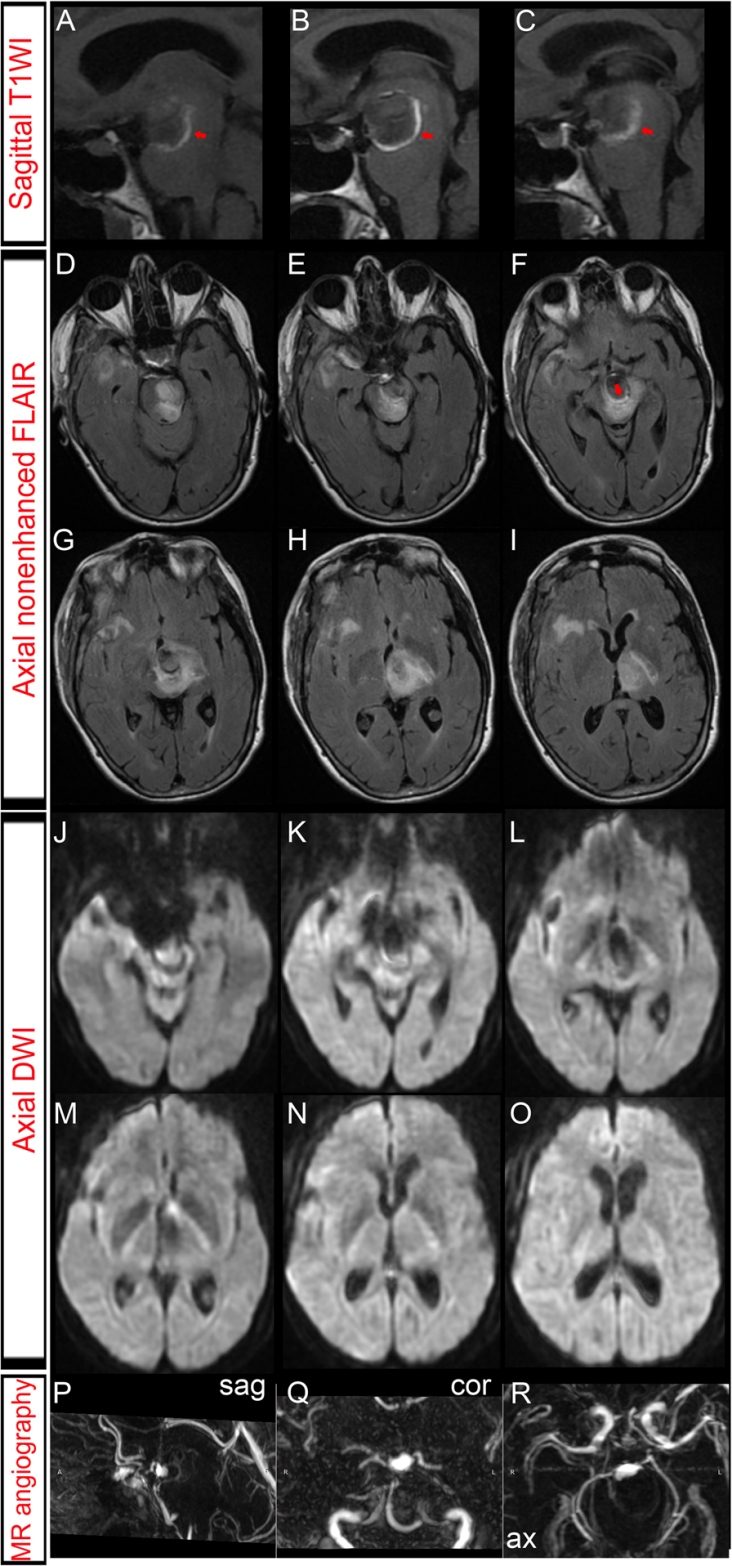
Postoperative magnetic resonance imaging (MRI) did not detect ischemic complications. a-c: Sagittal nonenhanced T1-weighted images (T1WI) exhibited a newer clot, expressed as a sickle-shaped high signal intensity area (red arrows), on the outer surface of the intraluminal old thrombus. d-i: Fluid-attenuated inversion recovery (FLAIR) MRI depicted a thrombosed giant aneurysm severely compressing the brainstem in the backward direction. In addition, we observed marked edema surrounding the basilar apex (BA) aneurysm and involving the midbrain, thalamus and left internal capsule. The sickle-shaped high signal intensity structure was also observed on FLAIR images (red arrow in F). j-o: Diffusion weighted imaging (DWI) showed no high-intensity lesions, thus excluding ischemic complications. p-r: Sagittal (sag), coronal (cor) and axial (ax) maximum intensity projection (MIP) reconstructions of magnetic resonance angiography (MRA) showed a > 50% decrease of the aneurysm blood lumen
